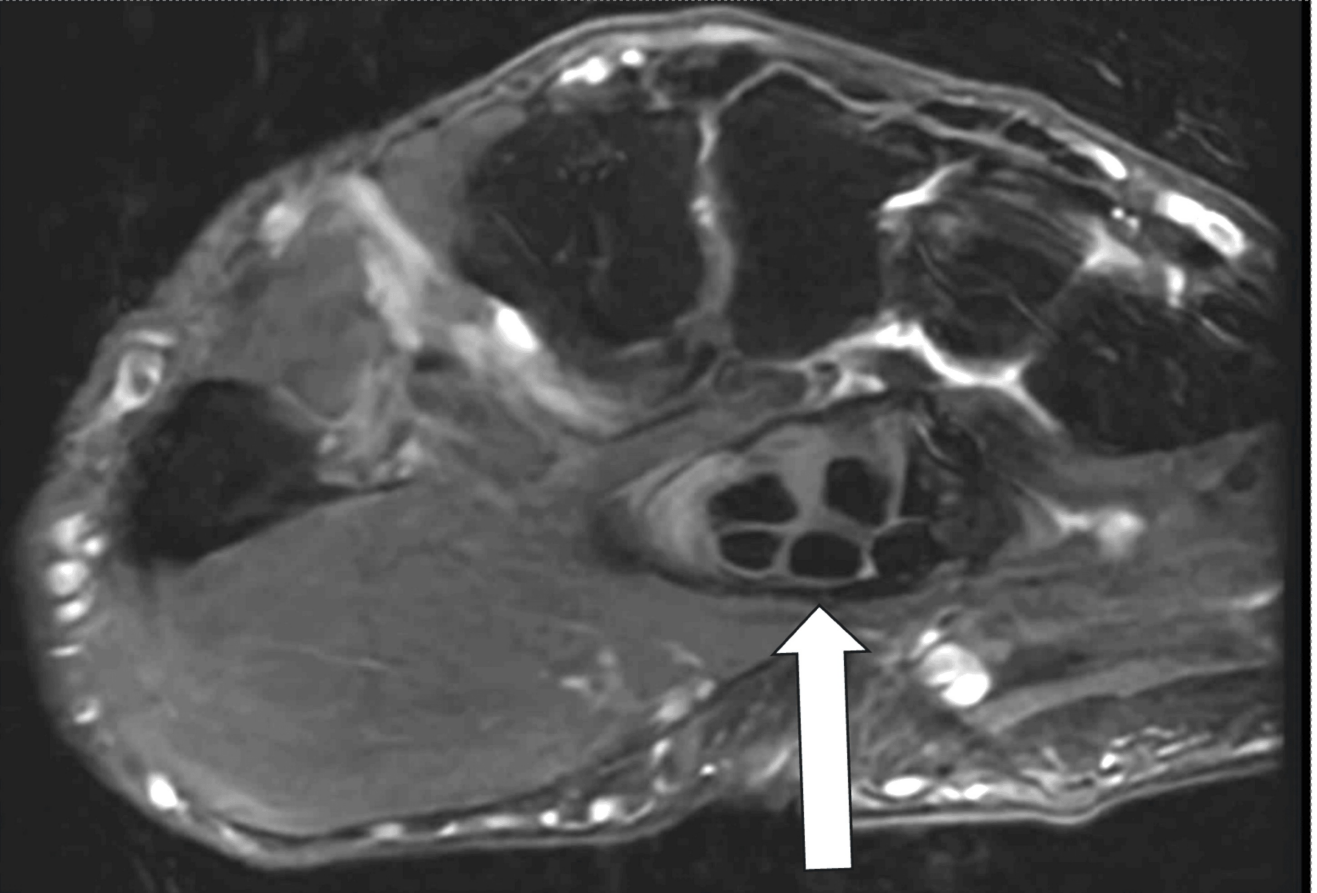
Axial T2 MRI of the wrist at the level of the third metacarpal base that demonstrates the palpable landmark (the distal edge of the transverse carpal ligament) in relation to the third metacarpal base. White arrow = distal edge of the transverse carpal ligament.
